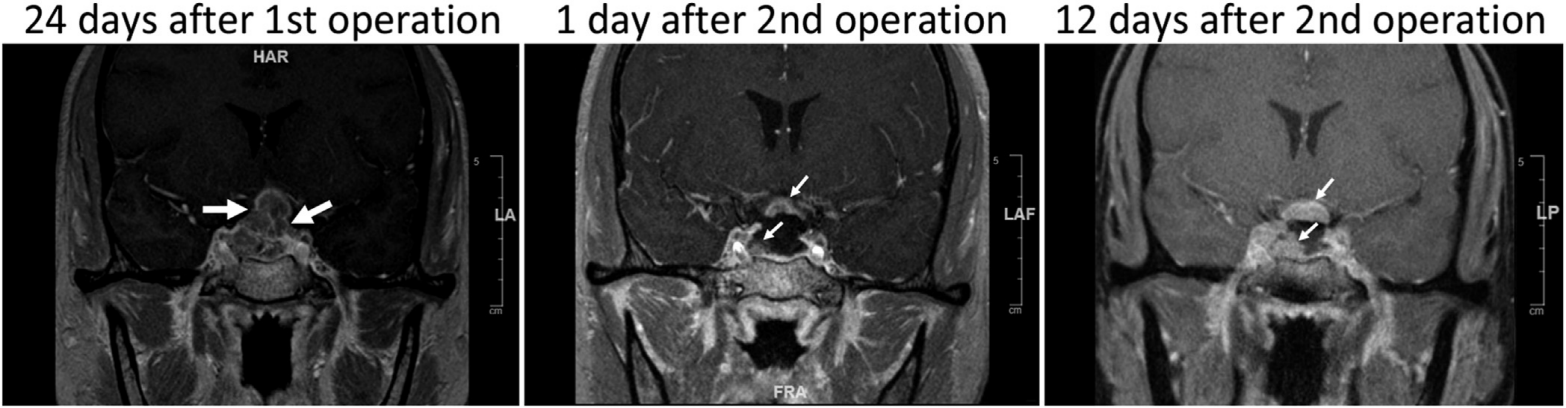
Pituitary magnetic resonance imaging of patient 2 after the first transsphenoidal operation. Left, 24 days after the first transsphenoidal operation. The tumor splayed and compressed the optic chiasm with associated edema of the optic tracts, optic chiasm, and prechiasmatic optic nerves. There was mass effect on the right cavernous sinus and dural thickening along the planum sphenoidale. Middle and right, 1 and 12 days after the second operation, respectively. During the 11 days, the residual tumor in the right sella grew from 8 mm × 6 mm to 18 mm × 11 mm, and the residual tumor in the suprasellar cistern involving the optic chiasm grew from 3 to 6 mm in maximum thickness. Arrows indicate the tumor.
